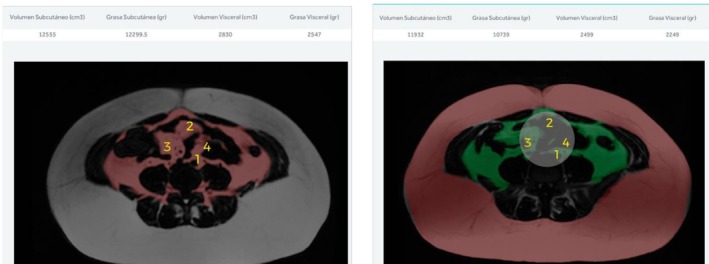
Cross‐sectional MRI image of a woman's abdomen showing observed fat loss, with a reduction of 1816 g in subcutaneous fat and 298 g in visceral fat. The points of greatest loss have been pointed out. The left figure indicates before and the right indicates after.
